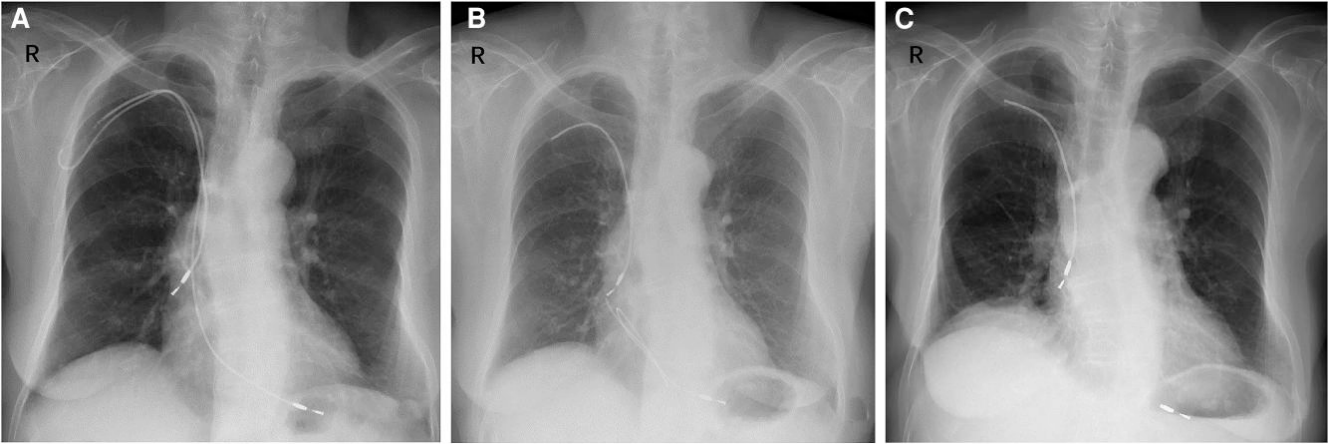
(*A*) The X-ray after the first debridement (the generator was removed, and the leads were embedded subcutaneously). (*B*) The X-ray after the second debridement (the exposed lead on the right subclavian area was cut off). (*C*) The X-ray after cutting the ventricular lead, removing the bacterial neoplasm under the tricuspid valve and performing the tricuspid valvuloplasty.
